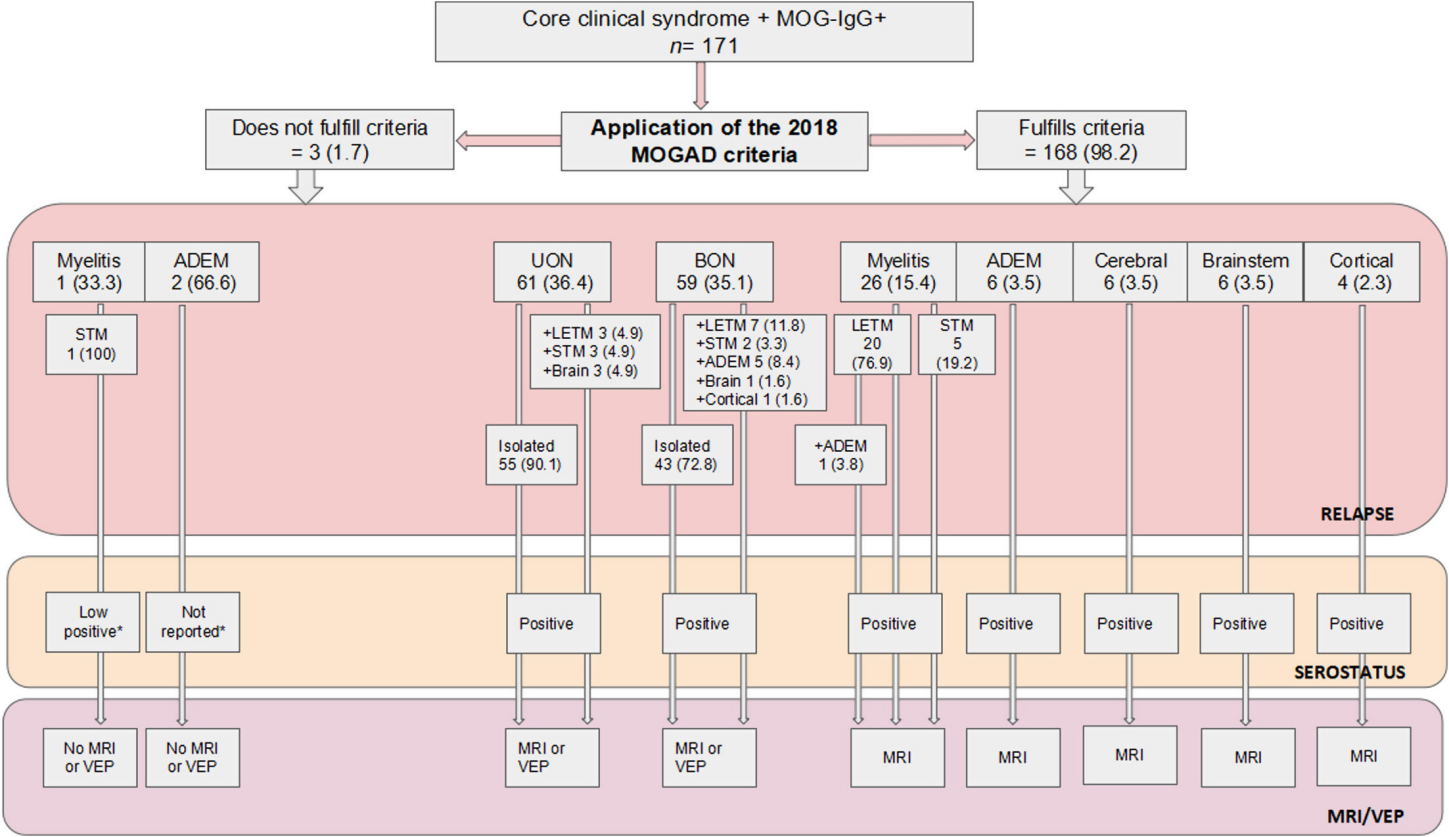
Application of 2018 myelin oligodendrocyte glycoprotein antibody (MOG‐IgG)‐associated disease (MOGAD) diagnostic criteria. All values are reported as absolute number and percentage. *In relation to MOG‐IgG titer. ADEM, acute disseminated encephalomyelitis; BON, bilateral optic neuritis; LETM, longitudinal extensive transverse myelitis; MRI, magnetic resonance imaging; STM, short transverse myelitis; UON, unilateral optic neuritis; VEP, visual evoked potentials.
